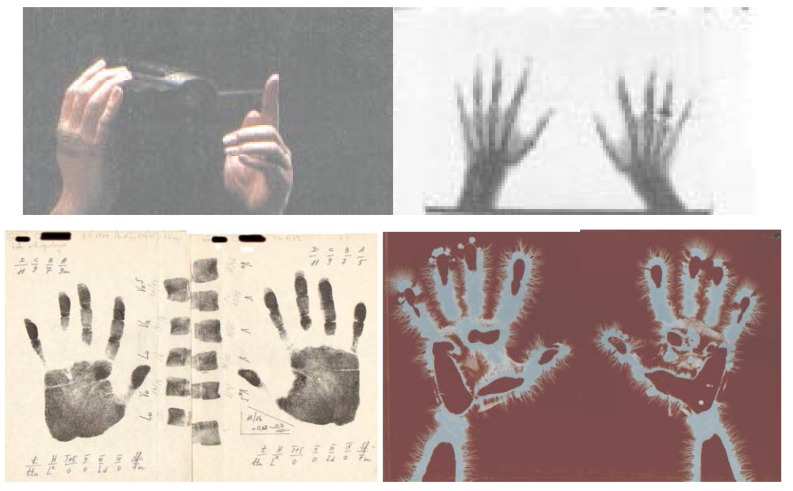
Four categories of investigation of the upper limb extremities: a) photographic image, b) radiographic image, c) dermatoglyphs (palmar prints), d) electrographic image (electrographic prints).
